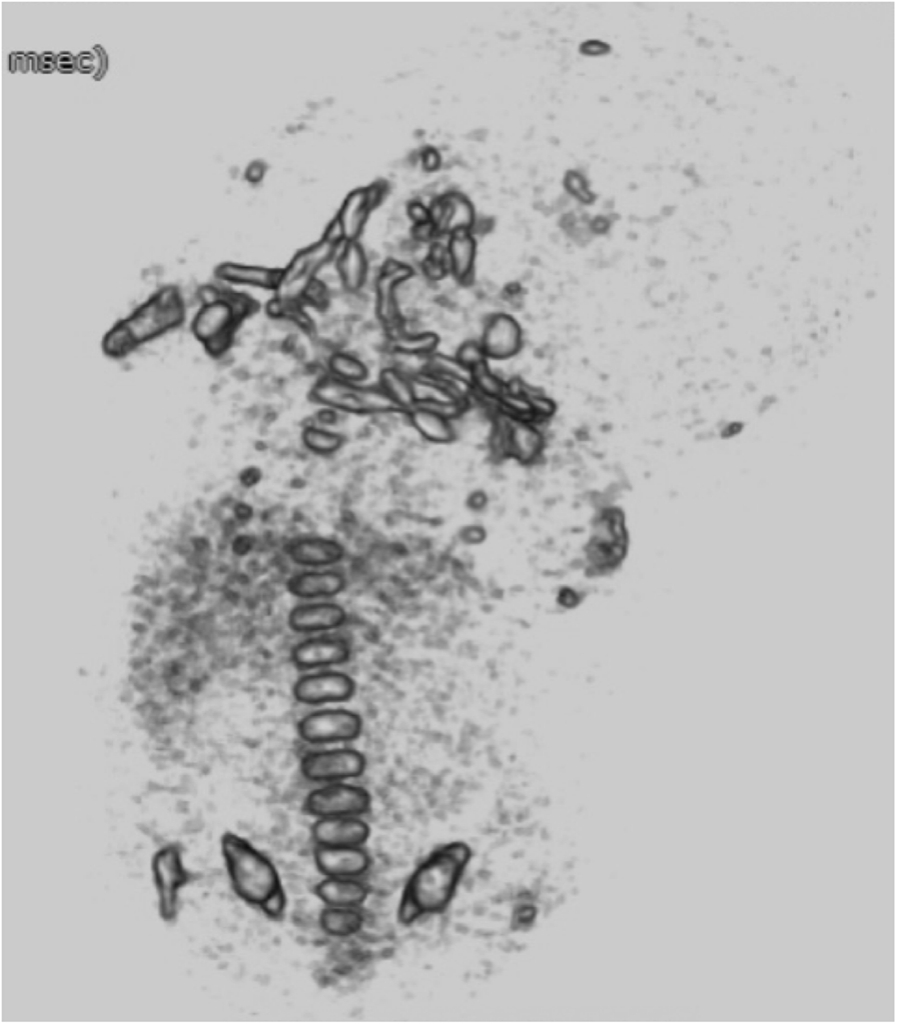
Fetal 3-dimensional computed tomography imaging of Infant 17: A case of hypophosphatasia
